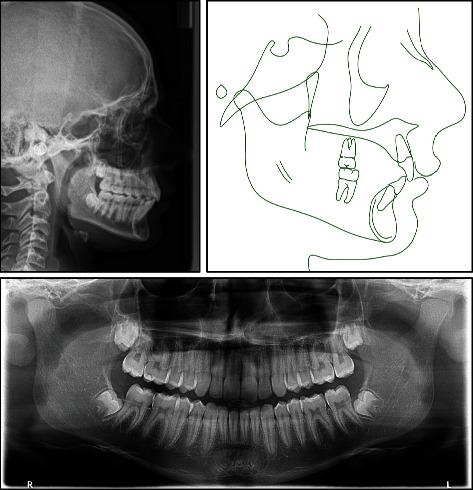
1-year follow-up radiographs and tracing.
